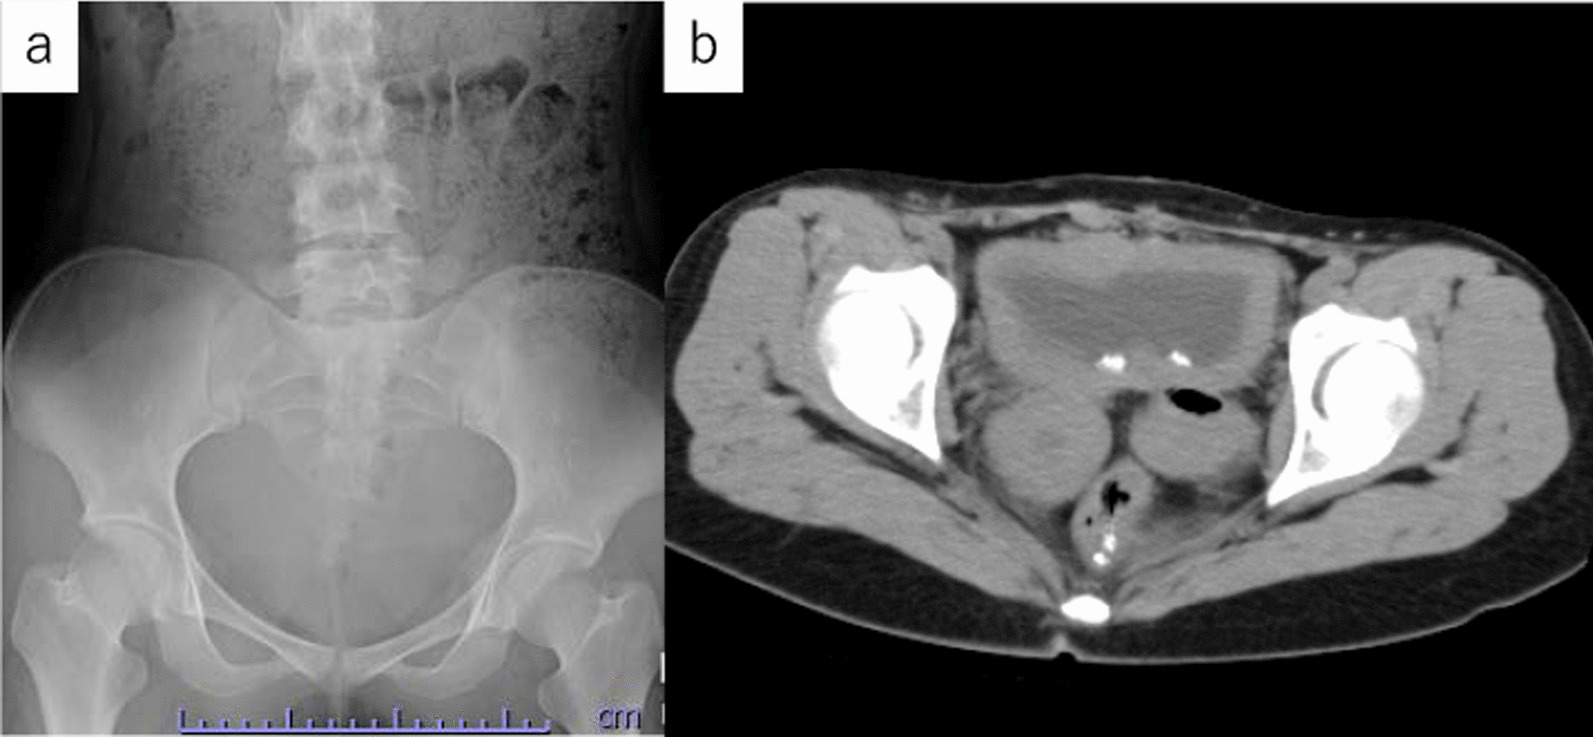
Postoperative a KUB and b CT
